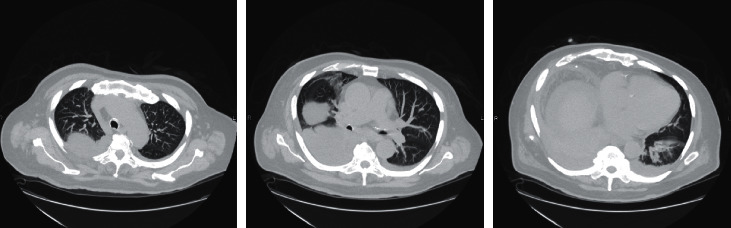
Computed tomography of the chest, showing a large, loculated effusion on the right suspicious for empyema.
